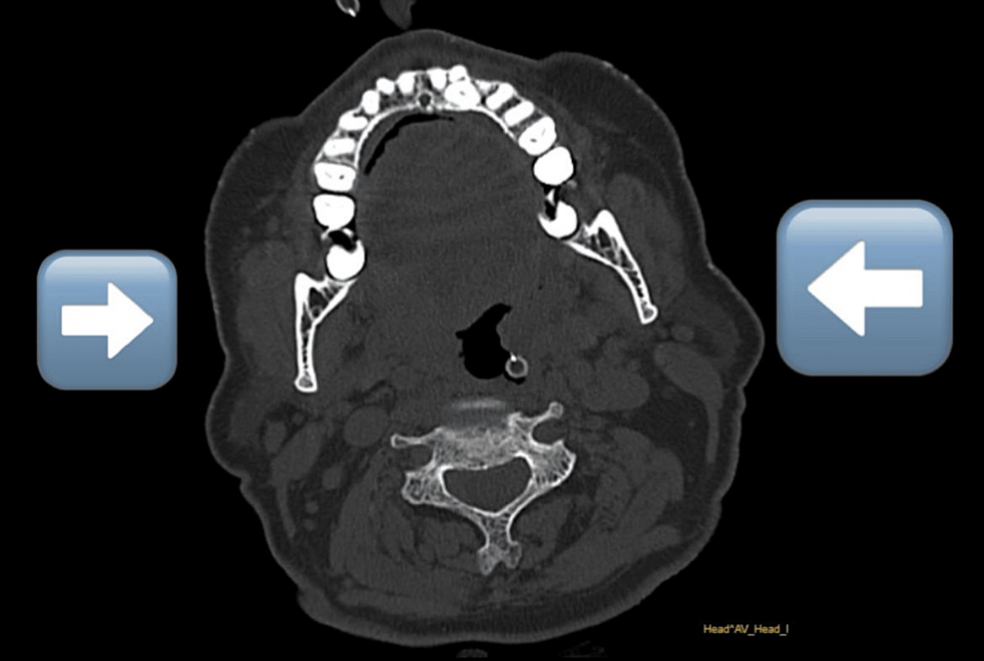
CT brain reveals diffuse irregular tortuous and narrow lumen with inhomogeneous signal seen involving the intracranial portion of bilateral internal carotid arteries.
